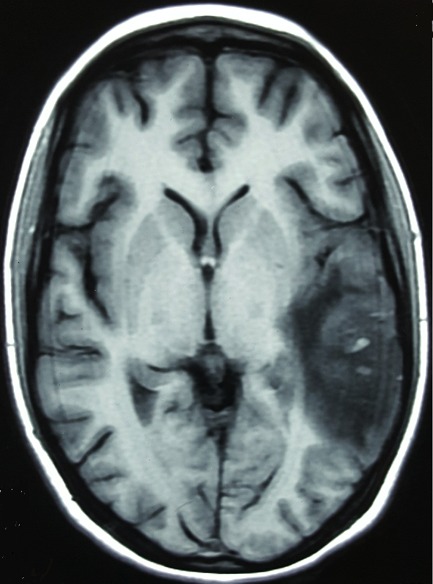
Magnetic resonance imaging. Contrast enhanced Axial T1 image showing a temporoparietal hypointense area with few petechial lesions
